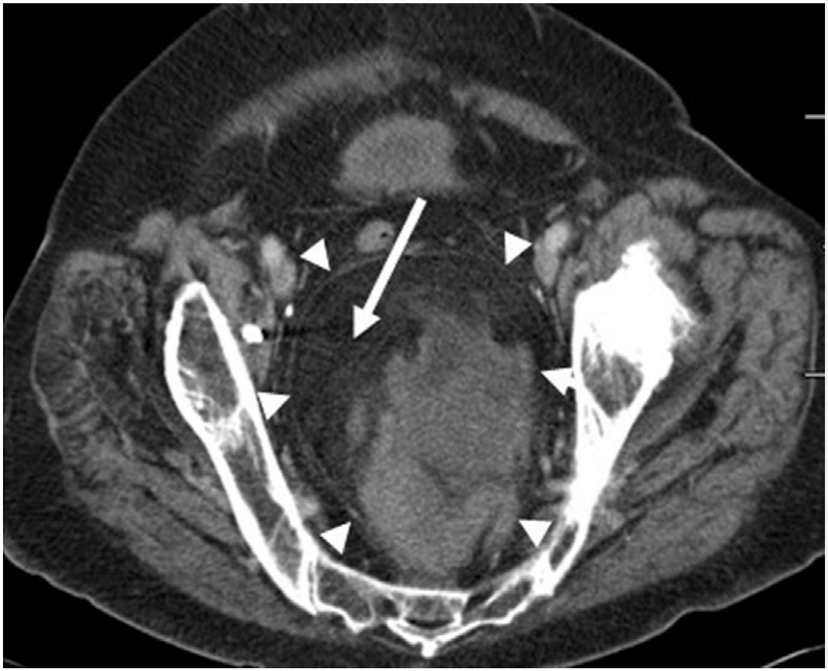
Axial CT image of the pelvis demonstrates a large fat containing mass within the presacral region (arrowheads). Fat within the mass is dark on the CT images (arrow).
